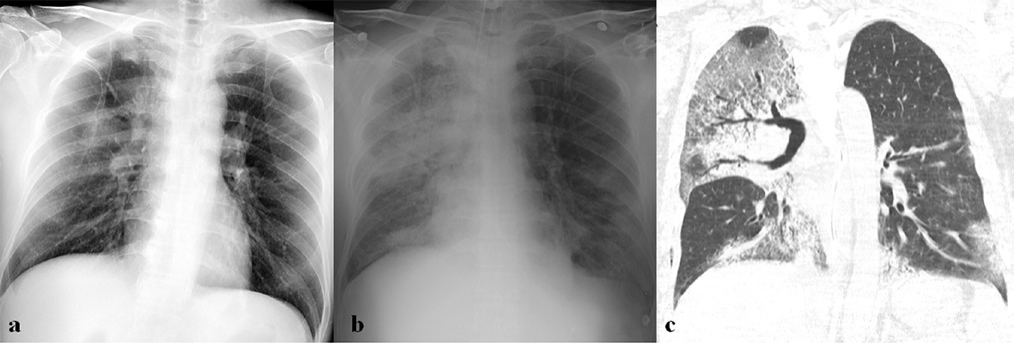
A 60-year-old male patient with MERS-CoV infection. (a) Chest radiograph (CXR) obtained on 8 days after exposure shows consolidation and GGOs in right upper lung zone. (b) Follow-up CXR obtained 18 days after exposure shows multifocal consolidations in both lungs. (c) Follow-up chest CT obtained on the same day with (b) shows bilateral, multifocal, mixed consolidation and GGOs with crazy paving appearance, suggesting organizing pneumonia.
